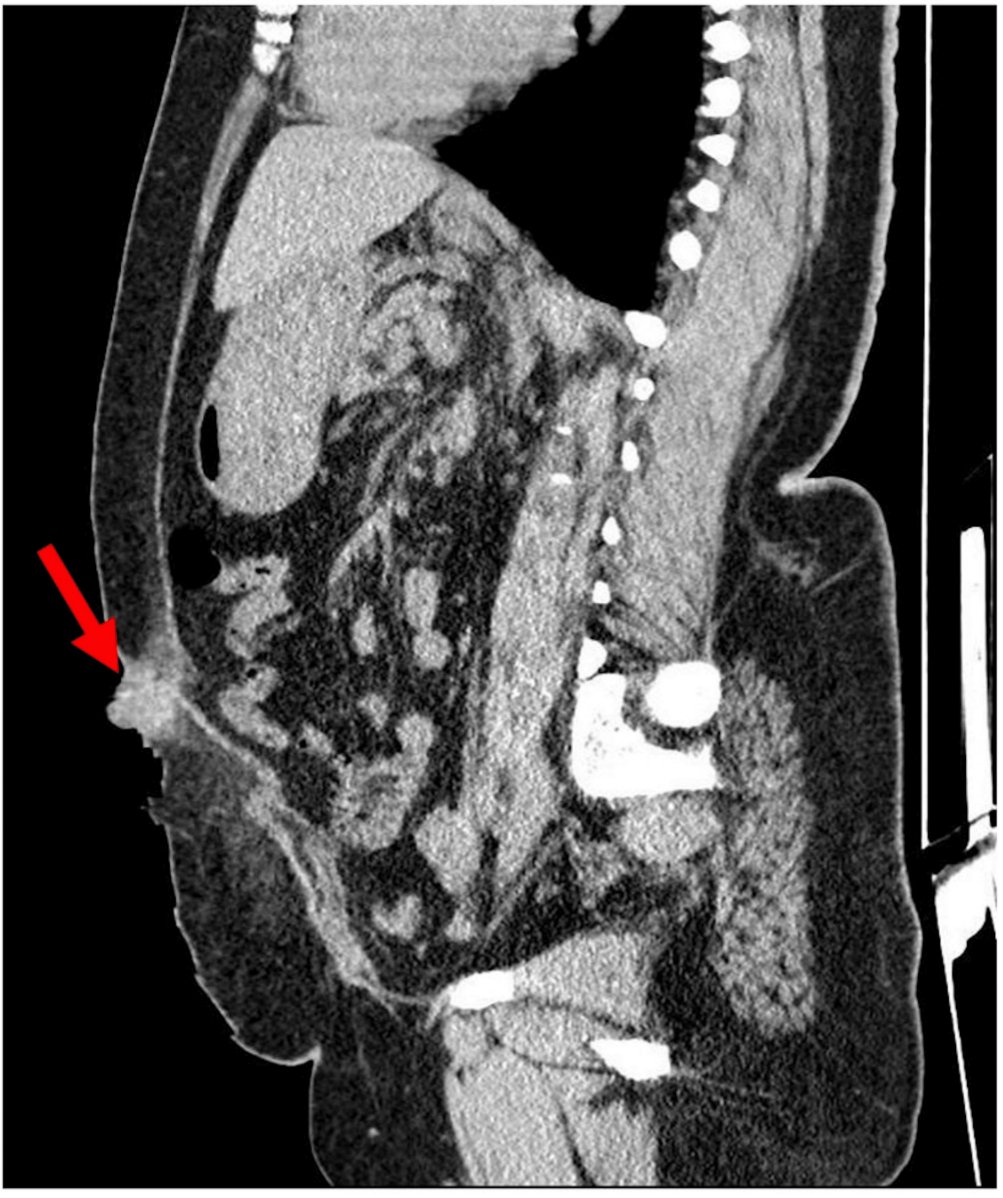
Sagittal-view CT scan revealing the umbilical lesion
